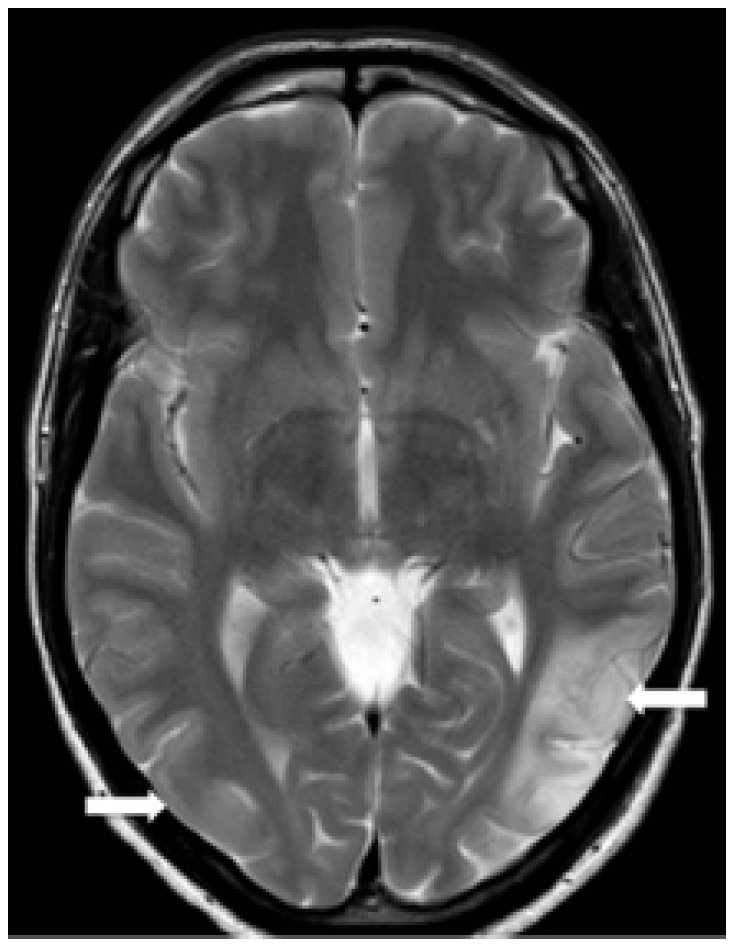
T2-weighted image reveals asymmetrical areas of hyperintensity, more marked in the left temporal region (white arrows).
